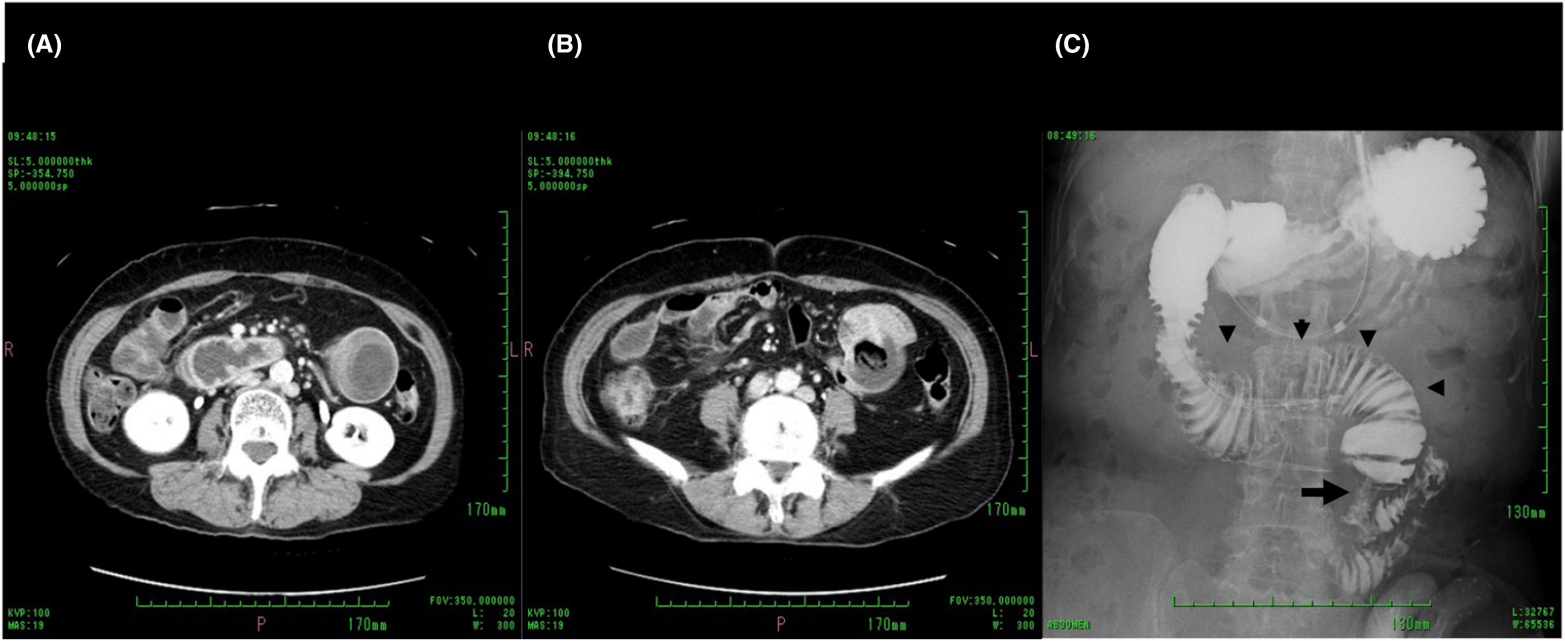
A contrast‐enhanced computed tomography image of the abdomen shows marked distension from the third portion of the duodenum to the jejunum around the ligament of Treitz (A). (A) circumferential tumor with heterogenous enhancement is shown at the upper jejunum (B). A contrast study through the nasojejunal tube shows localized stenosis of the upper jejunum (C, arrow) and dilation of the proximal intestine (C, arrowhead).
